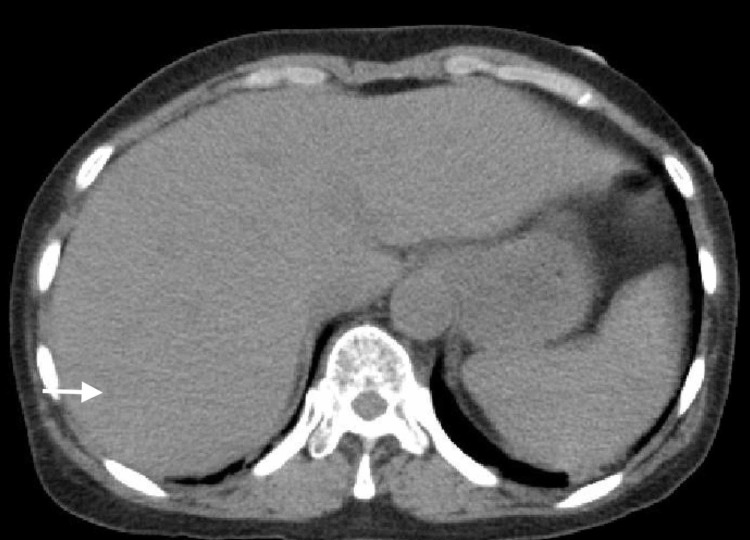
Computed tomographic scan of the abdomen showing mild hepatic steatosis
